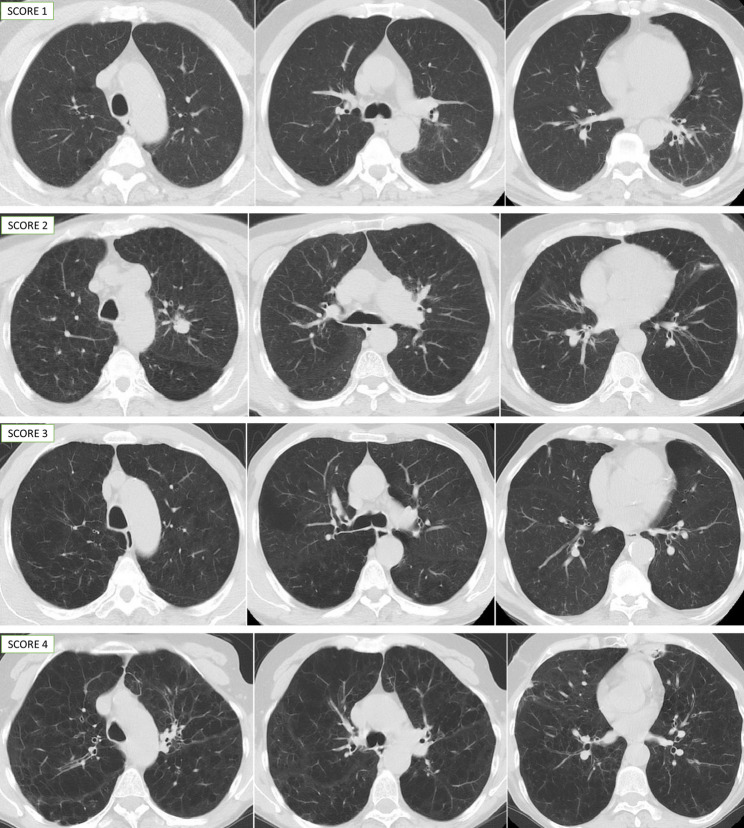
Example thoracic CT images of emphysema scoring
